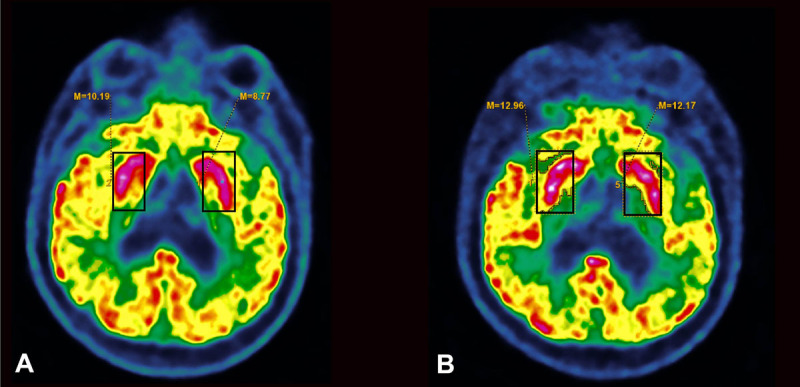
[18F]-fluorodeoxyglucose-positron emission tomography (FDG-PET) of the patient. A: FDG-PET before corticosteroid treatment, showing increased metabolism of the right putamen as compared to the left side (standardized uptake value (SUV) of 10.19 on the right side versus 8.77 SUV on the left, i.e. a difference of 16.2%); B: FDG-PET after corticosteroid treatment, showing resolution of the right putamen increased metabolism (12.96 SUV on the right side versus 12.17 on the left side, i.e. a difference of 6.5%).
